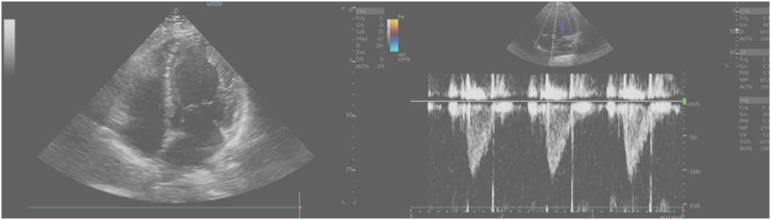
The patient’s echocardiogram upon initial admission.
